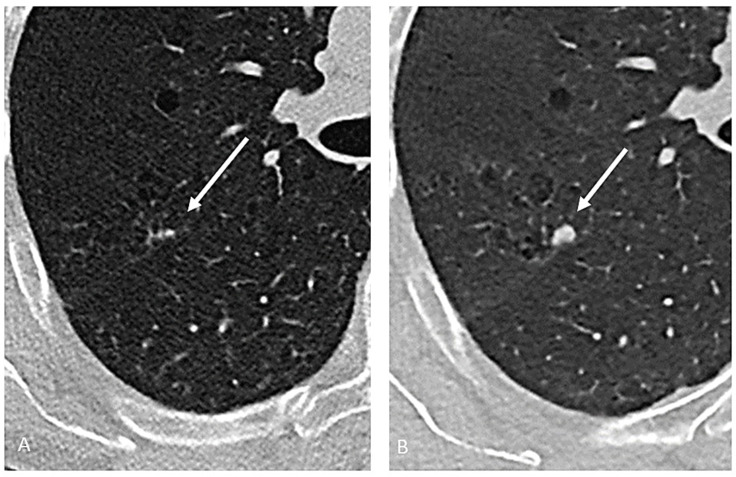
Axial chest CT showing a small area of increased density in the right superior lobe, adjacent to cystic emphysematous area (A), that increased in size and density after one year (B) and was proven to be an adenocarcinoma.
